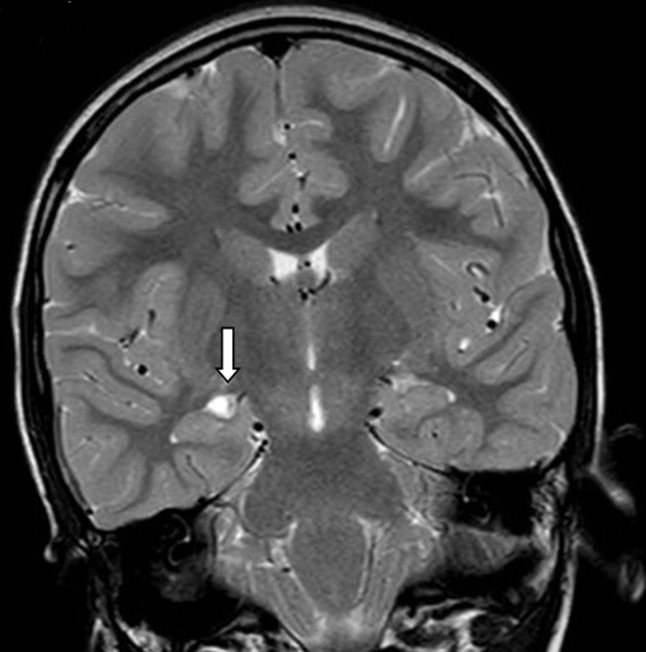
coronal MRI T2: same lesion, hypersignal T2 (arrow)
